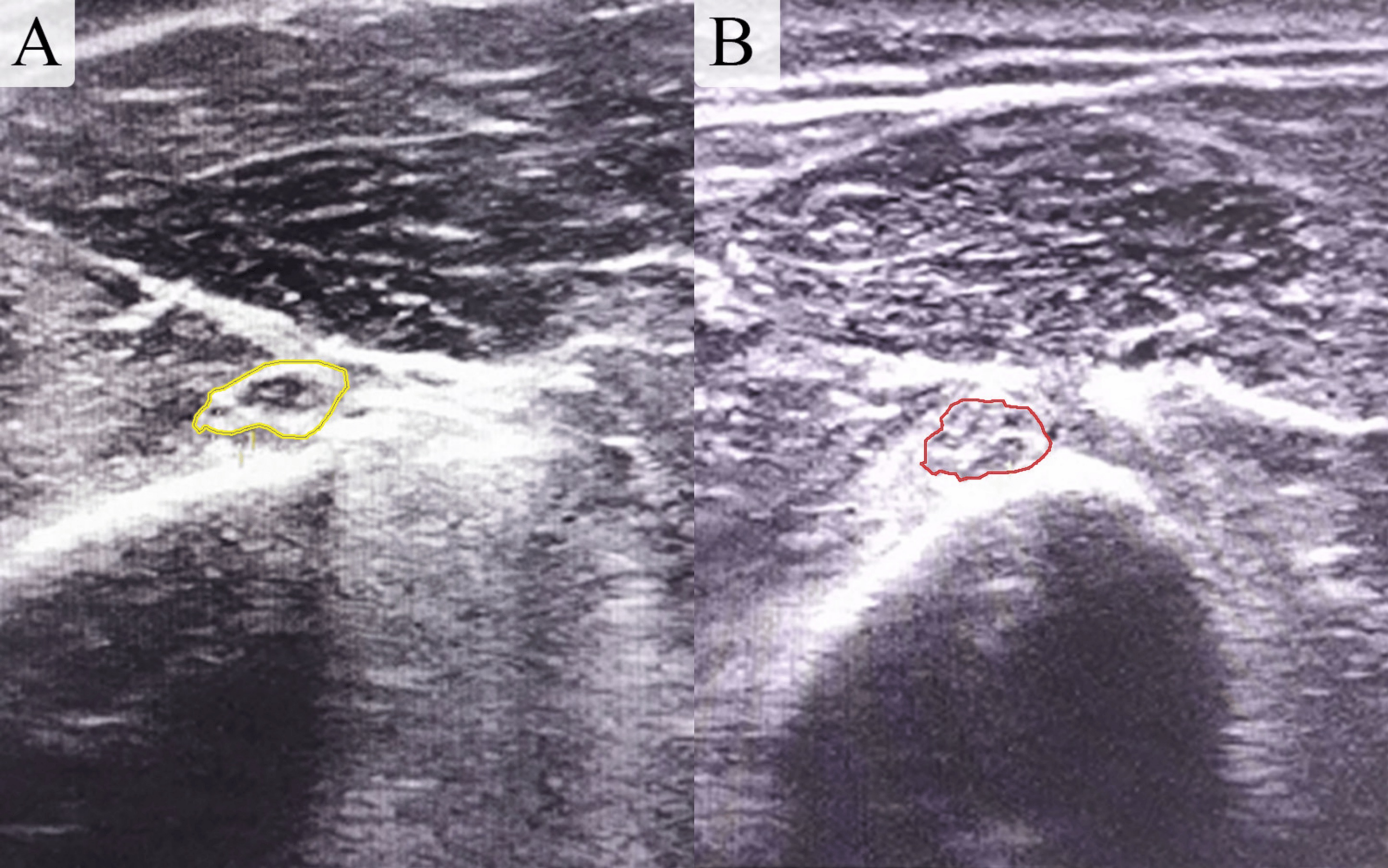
Comparative ultrasound images show a reduction in swelling and cross-sectional area (CSA) of the nerve, which decreased from 0.22 cm² at the time of the first injection (image B) to 0.11 cm² three months later (image A).
